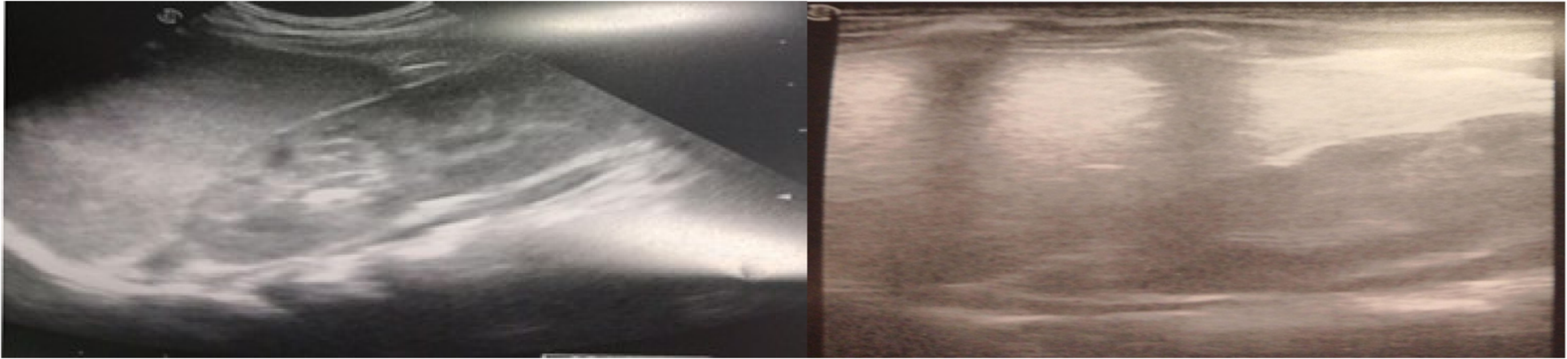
Complete regression of adrenal hemorrhage after 88 days of life with normal renal and adrenal ultrasound appearance
